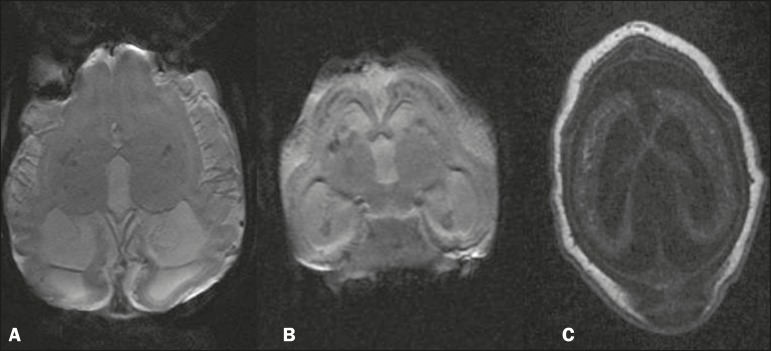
MRI. A,B: Volumetric gradient-echo sequences showing foci of signal
